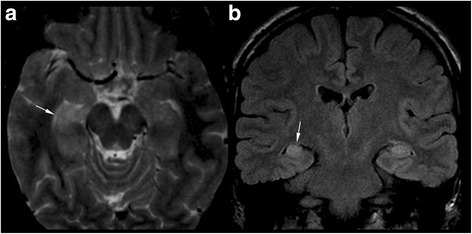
Magnetic resonance imaging of patient 1. a An axial FST T2-weighted image and (b) coronal FLAIR image of patient 1 shows slightly asymmetric hyperintense hippocampi (typical UBOs). The right hippocampus shows head volume loss (arrow) and flattened undulations (arrow in a) compared to the left thickened hippocampus. This is consistent with sclerosis
